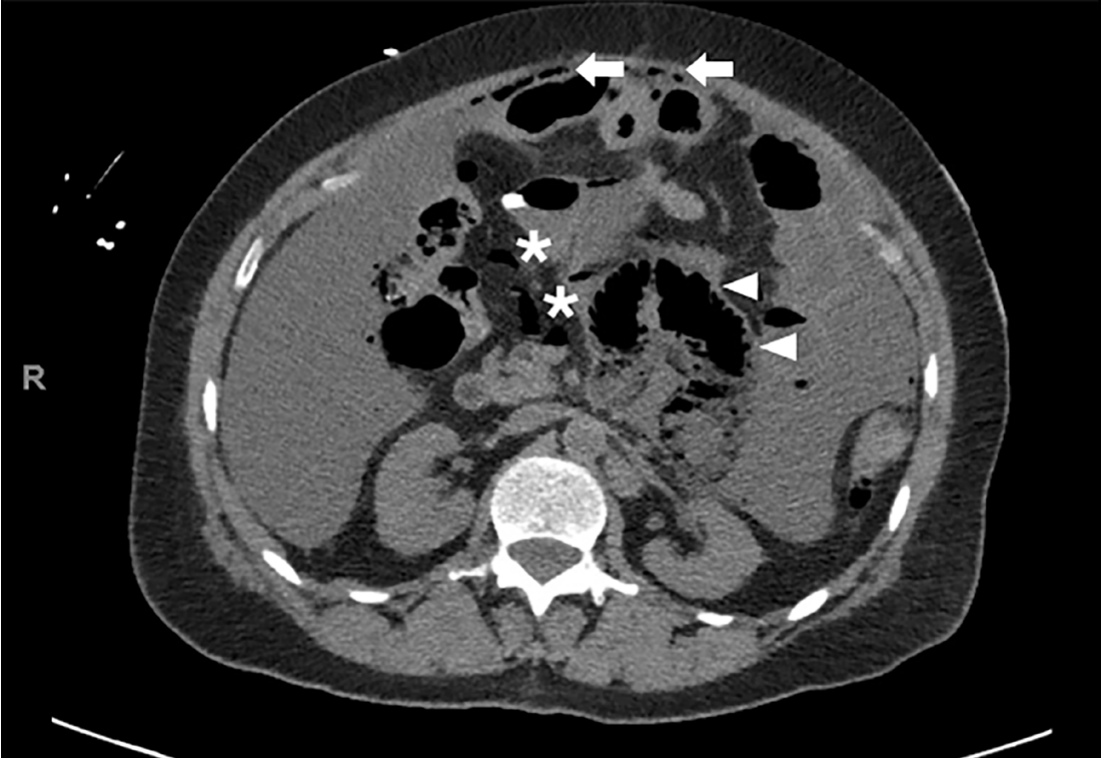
Computed tomography of the abdomen revealing intraperitoneal free air (arrows), bowel wall pneumatosis (triangles), and air in the mesenteric vasculature (*).
